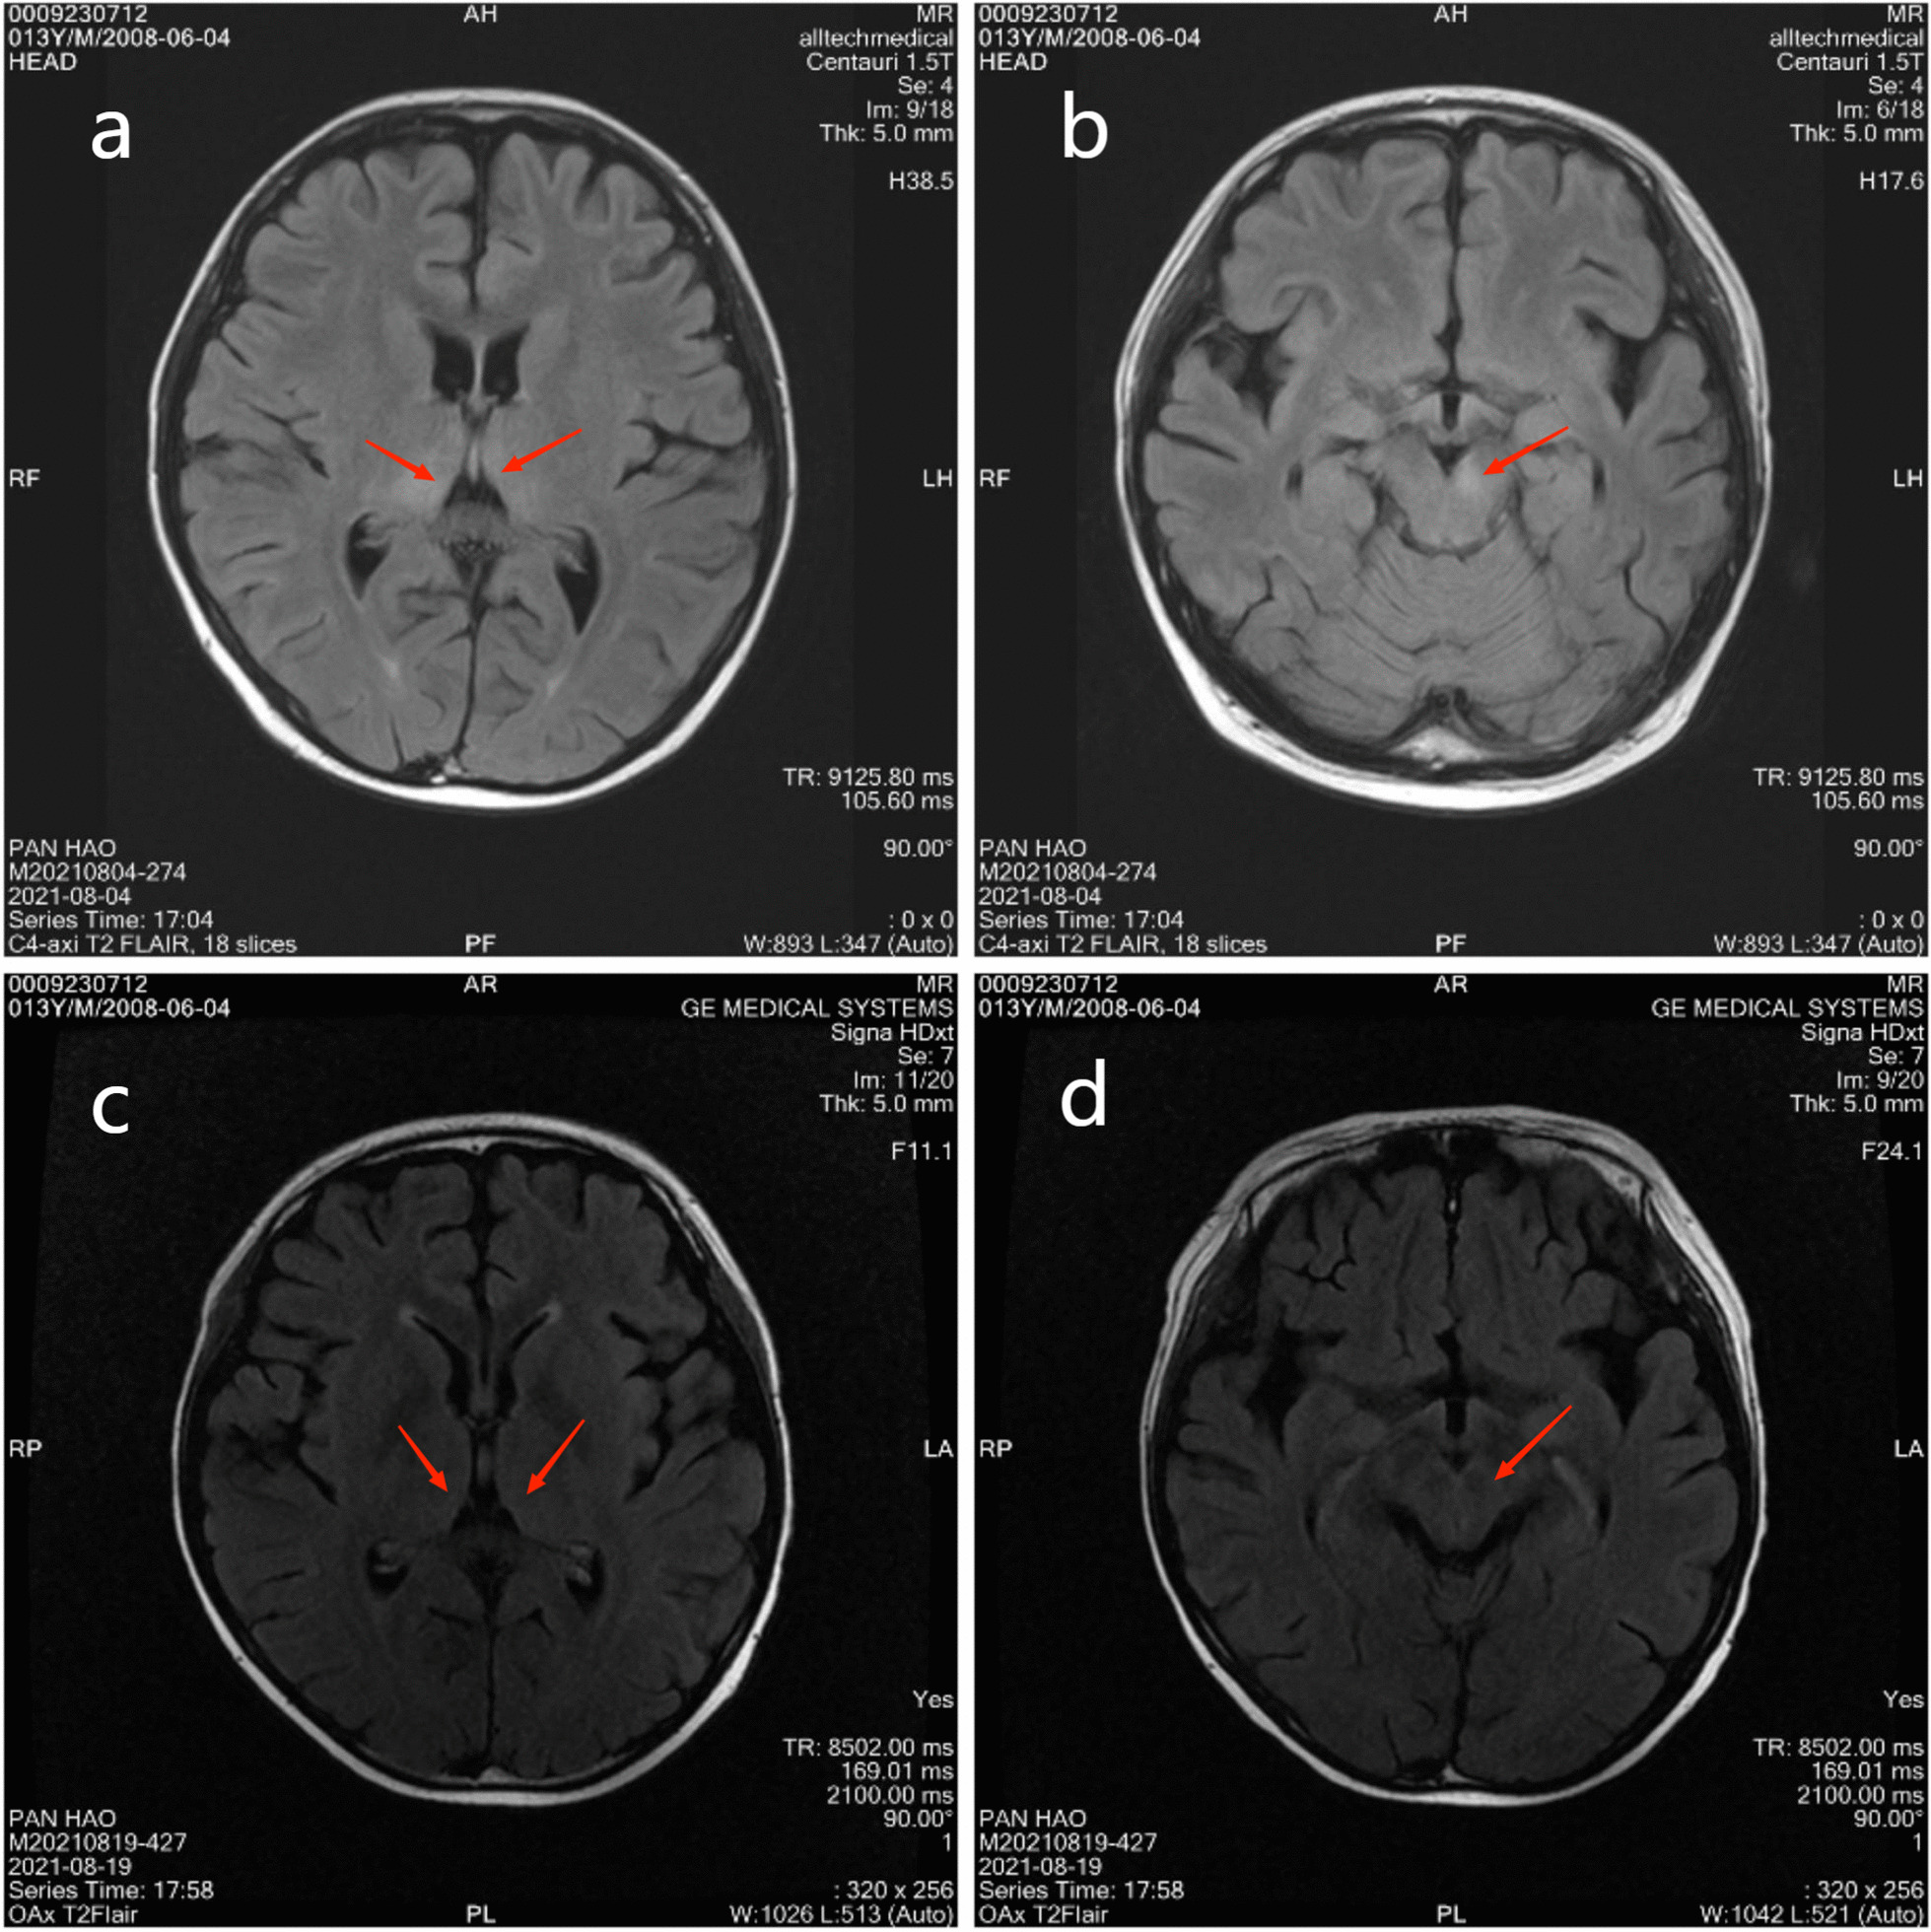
a–d Brain MRI revealed abnormal signal foci in the left midbrain cerebral peduncle and bilateral thalamus (red arrow)
